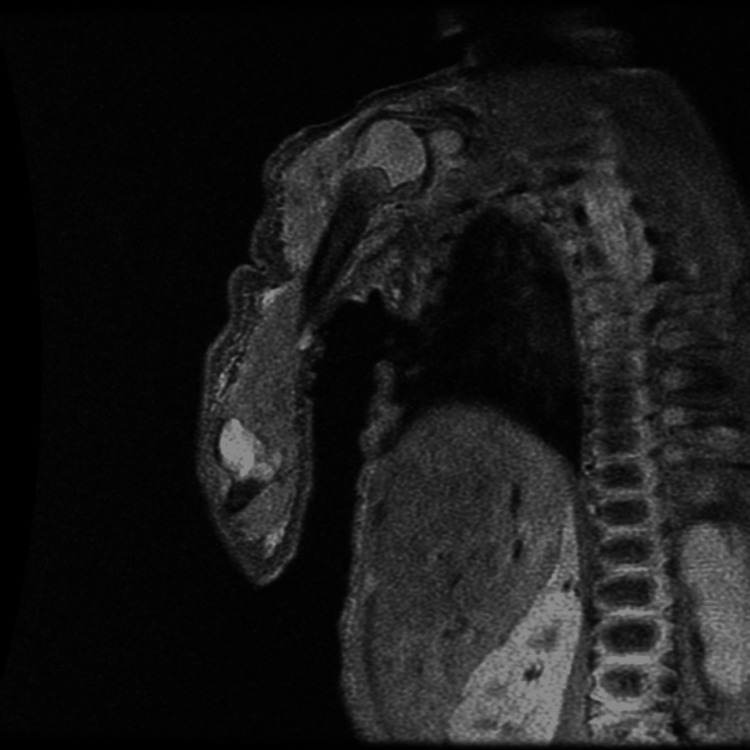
Coronal T2-weighted (STIR) right upper limb MRI demonstrating extensive inflammatory process on the shoulder. The image reveals marked, diffuse high signal intensity involving the soft tissues of the shoulder region, indicative of edema and myositis. Intra-articular hyperintensity is also noted within the glenohumeral joint, consistent with effusion.
